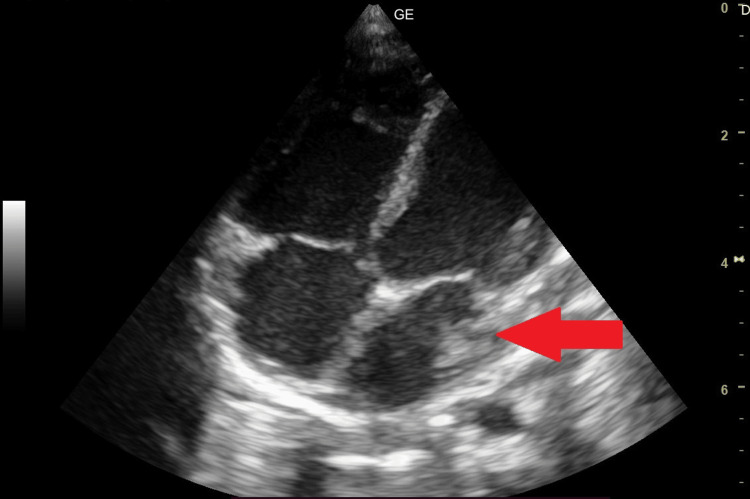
Ultrasound showing a rare association of intracardiac appendage in the left atrium attached to the atrial wall.
